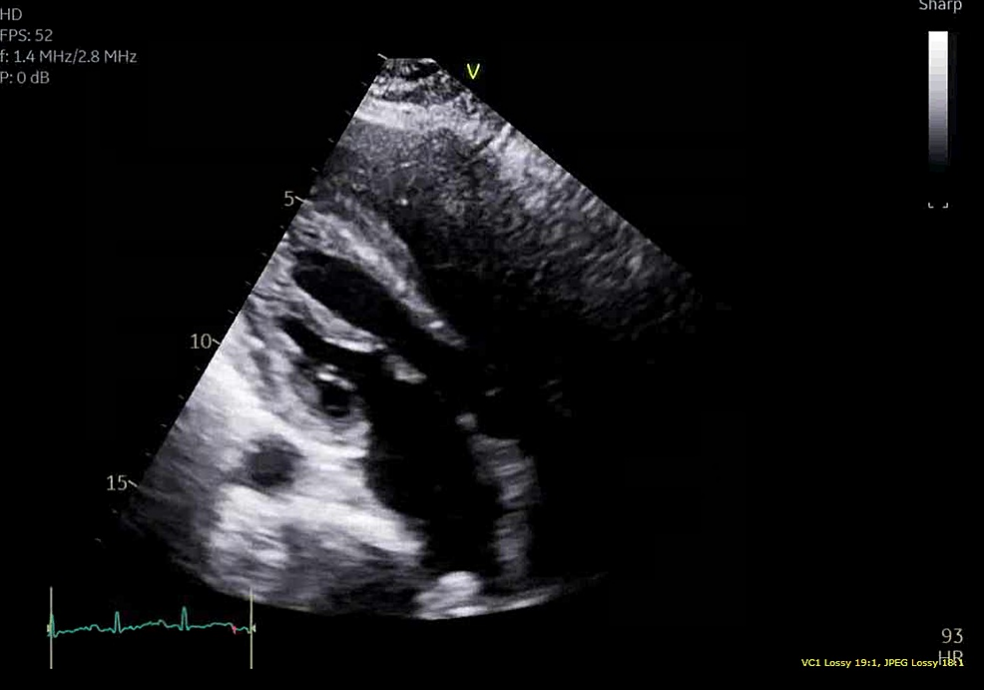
Transthoracic echocardiogram Findings of transthoracic echocardiogram consistent with hyperdynamic systolic dysfunction and increased flow across the left ventricular outflow tract.
